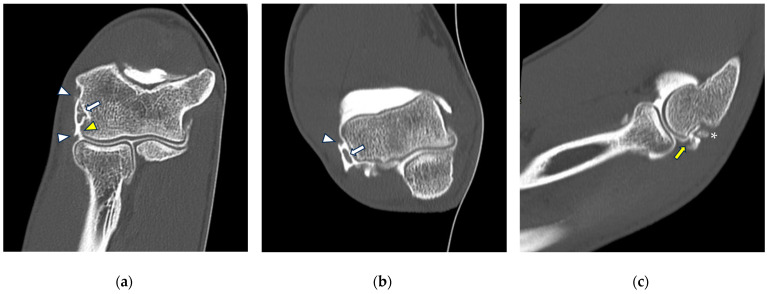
CT arthrography of a patient with sequelae of traumatic elbow dislocation. (a,b) Coronal and axial images showing LCL laxity with subtle foci of contrast medium permeation due to ligament delamination (white arrowheads) and a loose body within a pathologically distended lateral recess (white arrow), as well as cartilage thinning and fraying of the lateral aspect of the capitulum humeri (yellow arrowhead). Post-traumatic deformity of the radial head can also be seen; (c) sagittal reformat shows a loose bony fragment posterior to the capitulum humeri (asterisk) and posterior synovial thickening (yellow arrow).
